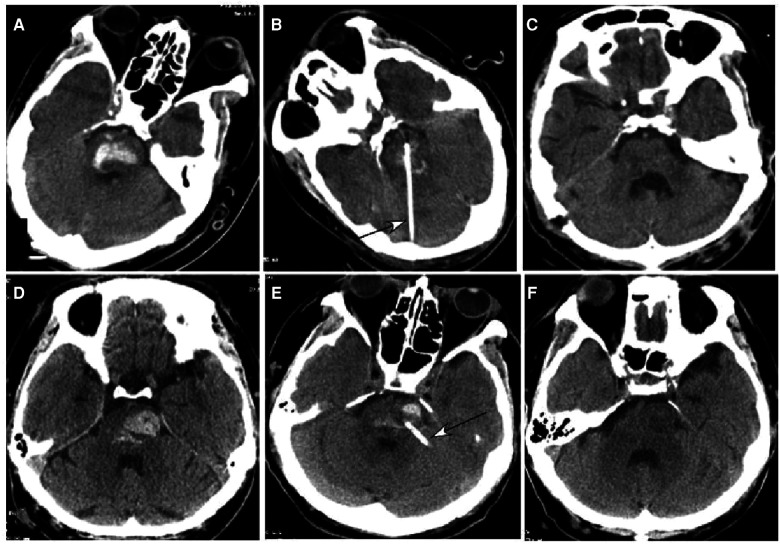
Brainstem hemorrhage. (A–C) A 52-year-old man had a sudden loss of consciousness 5 h before presentation. Before surgery, he was in a coma with a Glasgow Coma Scale (GCS) score of 5 (E1VTM4) supported with mechanical ventilation. (A) Computed tomography (CT) revealed brainstem hemorrhage. (B) Stereotactic aspiration was performed (arrow). (C) After surgical aspiration, the hematoma in the brainstem disappeared, and he became conscious with a GCS score of 10 (E4VTM6) and a Modified Rankin Scale score of 4. Twenty days later, he could move his hands. (D–F) A 46-year-old man had a sudden onset of unconsciousness with nausea and vomiting for three hours before presentation. (D) CT imaging demonstrated brainstem hemorrhage. Before surgery, he was in a coma with a GCS score of 5 (E1VTM4) and mechanical ventilation. (E) Stereotactic aspiration was performed (arrow). (F) Three weeks after the surgery, the hemorrhage in the brainstem disappeared, and he regained his consciousness with a GCS score of 10 (E4VTM6) and a Modified Rankin Scale score of 4.
